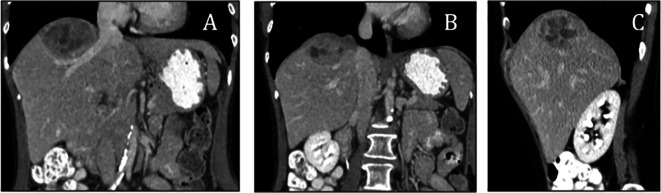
Computed tomography showed an arched lesion on the liver near the inferior vena cava (A-C).
